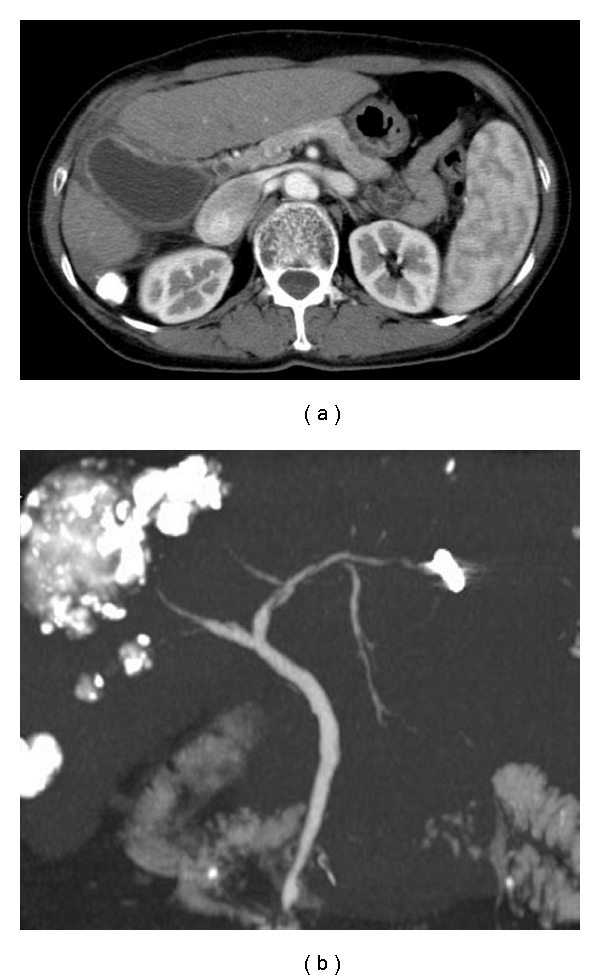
(a) Gallbladder swelling with wall thickness remained on abdominal CT 30 days after admission. (b) Gallblader and cystic duct were not visualized on three-dimensional spiral CT cholangiography.
